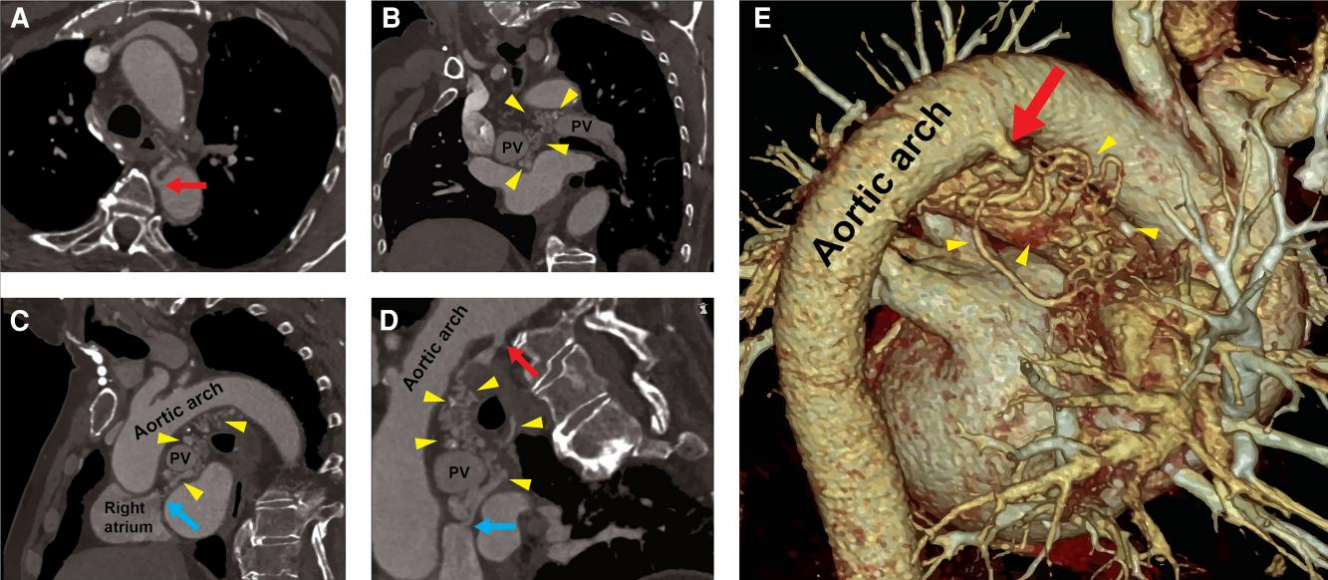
Computed tomography showing an overview of the mediastinum, revealing that the vessel visualized in the angiography was not a simple arteriovenous fistula but rather an arteriovenous malformation. *(A)* Axial view. *(B)* Coronal view. *(C)* Sagittal view. *(D)* A vessel tracking reconstruction of the arteriovenous malformation. *(E)* 3D reconstruction of the mediastinal arteriovenous malformation. Red arrow marks the origin of the arteriovenous malformation from the left bronchial artery. Blue arrow marks connection of the arteriovenous malformation to the origin of the right atrium. Yellow arrowheads mark the arteriovenous malformation. PV, pulmonary vein; AVM, arteriovenous malformation; CT, computed tomography.
